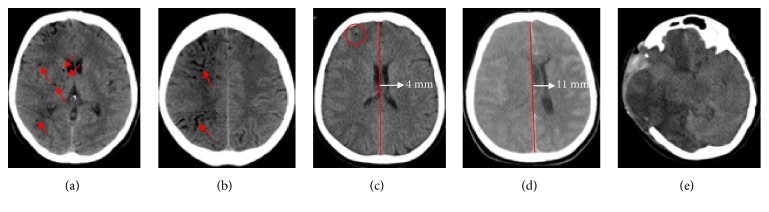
Head CT scan: (a) before HBOT: gas bubbles in the basal nuclei and (b) within the subarachnoid spaces (arrows); (c) immediately after HBOT: single right frontal bubble (circle) and 4 mm: leftward deviation of the midline (arrow); (d) 14 hours after HBOT 4 mm: leftward deviation of the midline; (e) 5 days after right decompressive craniectomy: massive brain herniation with right hemispheric and left frontal ischemia.
